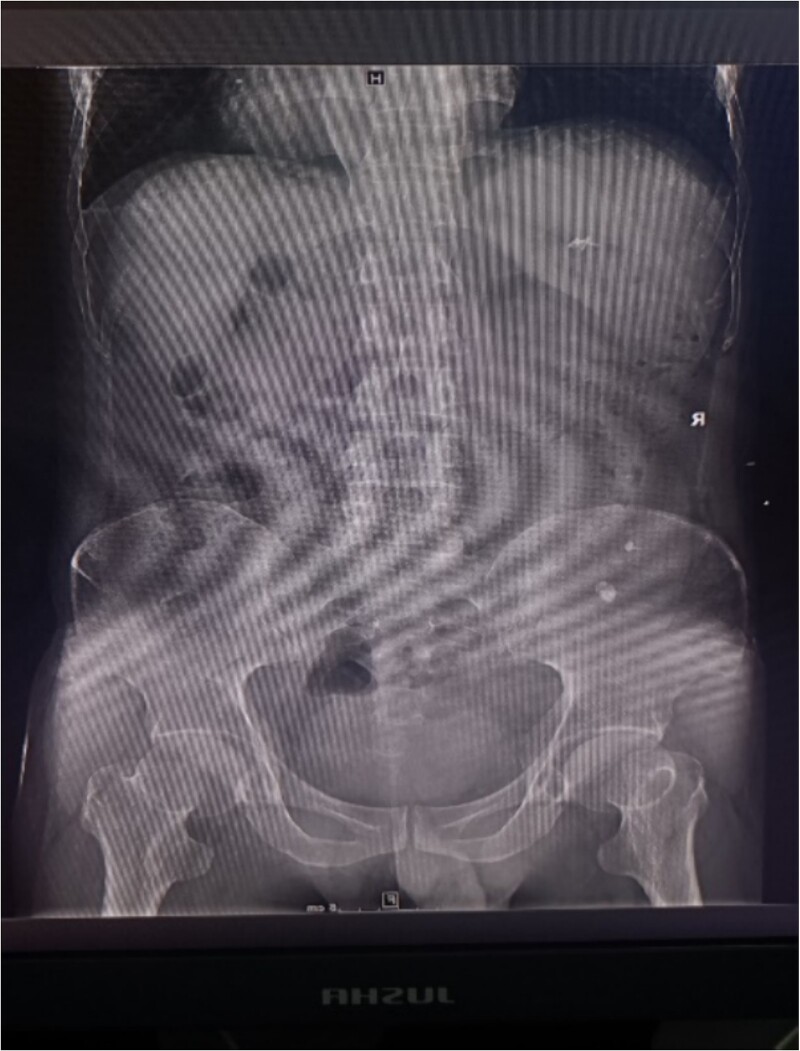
X-ray plain film showing multiple punctate stones in the right kidney area.
